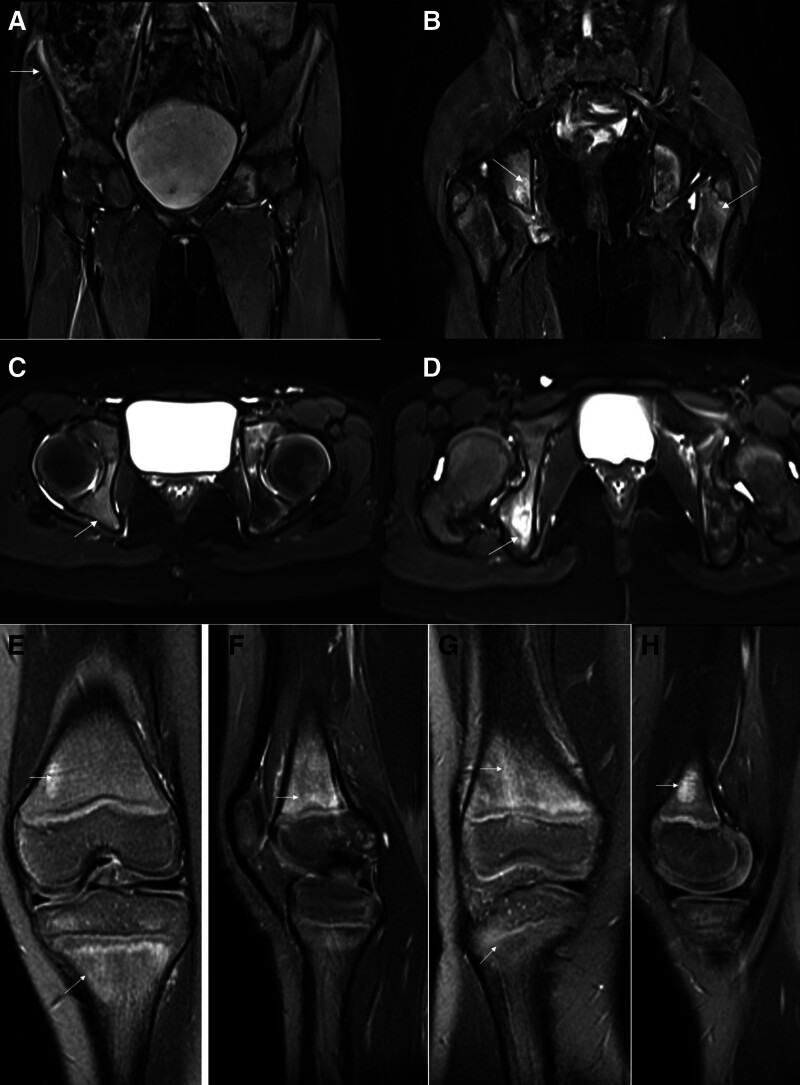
Abnormal signal intensity on MRI of the hips and knees of a 3-year-old girl. (A–D) Hyperintensity (white arrows) on T2-weighted images, involving the pelvis and femur; (E–H) T2-weighted fat-saturated MRI showed metaphyseal and epiphyseal osseous edema of the tibias and femurs (white arrows). MRI = magnetic resonance imaging.
